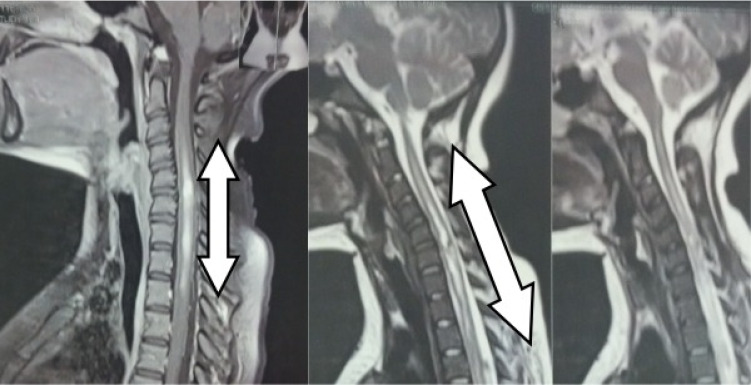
Spinal cord resonance showing longitudinally extensive transverse myelitis in cervical-thoracic segments (more than 3 vertebral segments). Sagittal section with T1 and T2-weighted acquisition.
